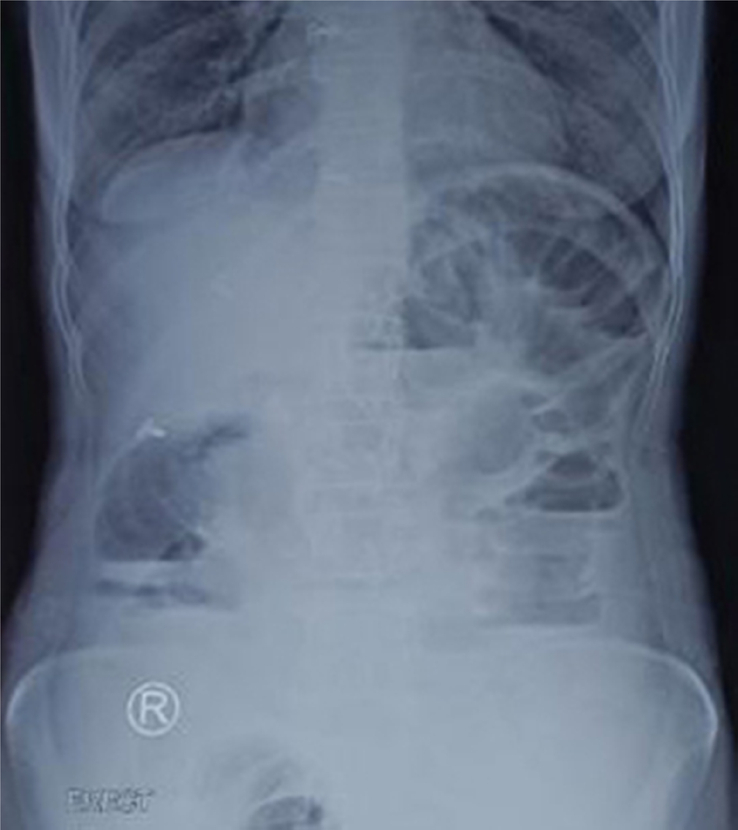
Plain abdominal X-ray showing multiple air-fluid levels and dilated bowel loop suggestive of small bowel obstruction.
